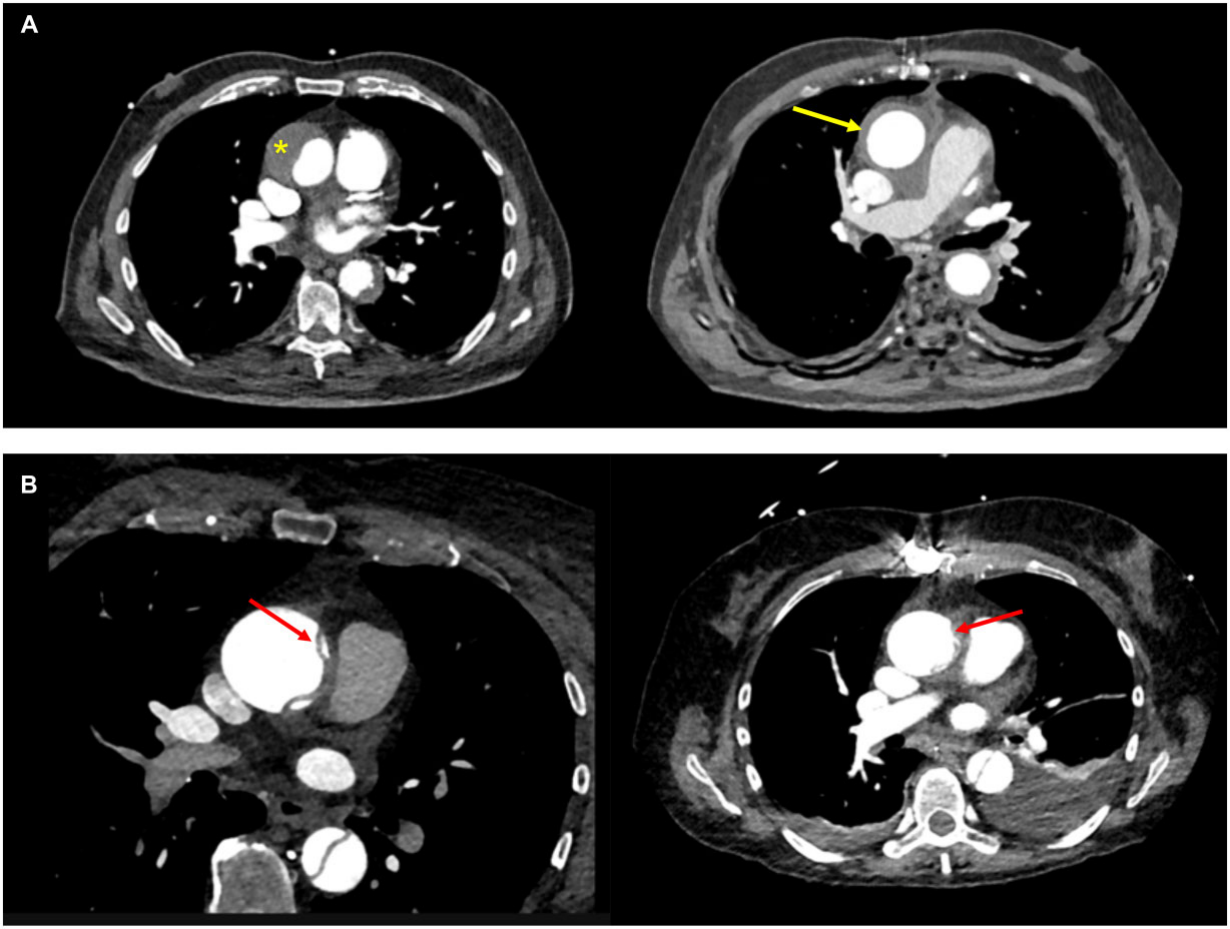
(A) Preoperative CT scan of the 87-year-old patient with an intramural haematoma of the ascending aorta (left) with a thickness of 22 mm (*) and postoperative control 3 months following wrapping (right): the intramural haematoma has practically completely disappeared (arrow) and the aortic diameter has remained stable. Retrospectively, it was suspected that the primary tear occurred in the proximal descending aorta and extended in a retrograde way up to the ascending aorta. (B) Preoperative CT scan in a 72-year-old female under oral anticoagulation demonstrating acute aortic dissection with the flap in the ascending aorta (left with red arrow) and postoperative control 12 months later (right) showing an almost disappearance of the dissecting membrane in the ascending aorta (red arrow) and absence of aortic growth, peri-aortic haematoma and/or pericardial effusion.
